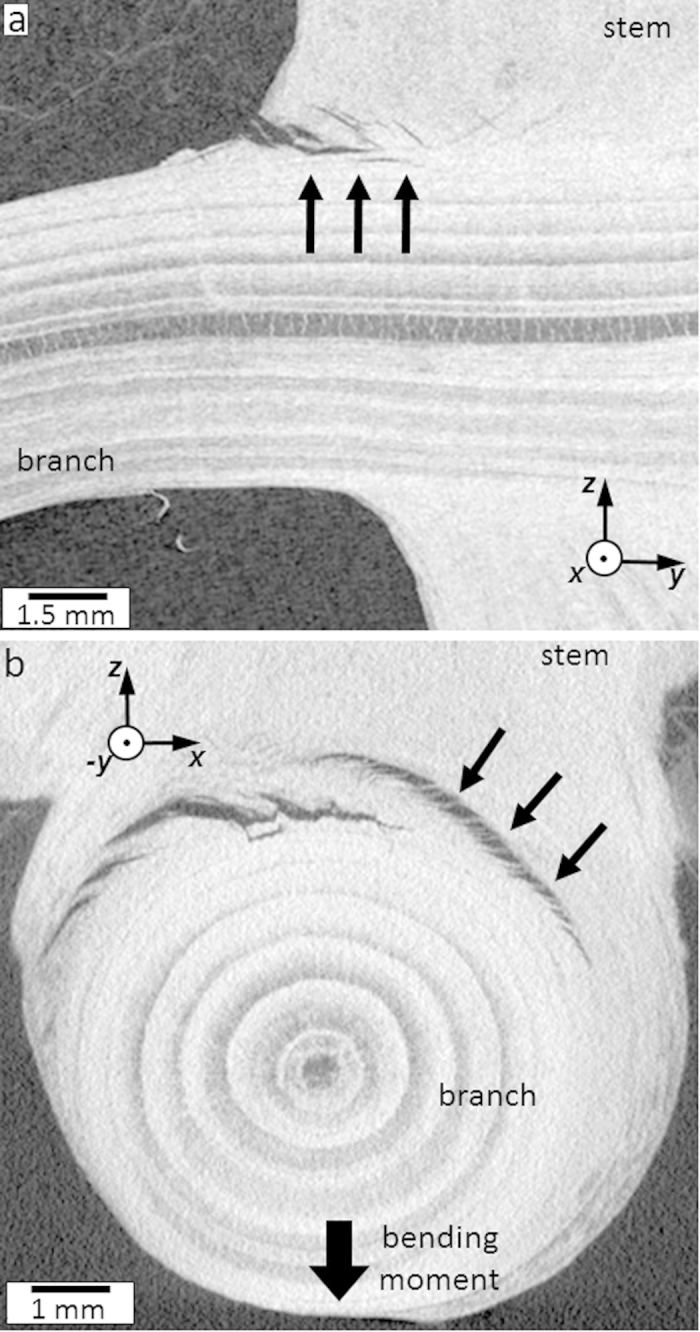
X-ray computer tomography images of a statically loaded branch-stem interface. (a) An early stage of the zig-zag crack pattern formation indicated by arrows is presented in the radial view. (b) The cracks are bridged by bundles of tracheids and wood rays of about 100 μm in thickness indicated by arrows in the axial view.
